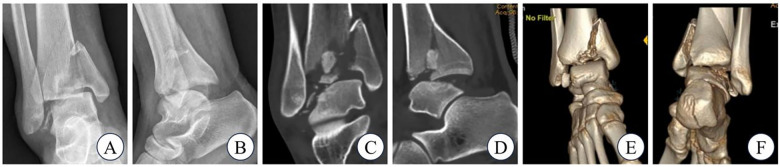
Patient: A 17-year old female was admitted 1 h after a fall from height, presenting with right ankle pain, deformity, and limited mobility. Diagnosis: 1. Right talar body fracture (Sneppen type I); 2. Right distal tibial fracture (AO/OTA 43B3, Rüedi–Allgöwer type II); 3. Right ankle subluxation; 4. Right lateral malleolar avulsion fracture. (A,B) Preoperative AP and lateral ankle radiographs revealing a fracture at the lateral dome of the right talus, a right tibial pilon fracture, and right ankle subluxation. (C–F) Preoperative CT 3D reconstruction: demonstrated a comminuted/compression fracture of the lateral talar dome, depression of the distal tibial articular surface, and the presence of a die-punch fracture fragment.
